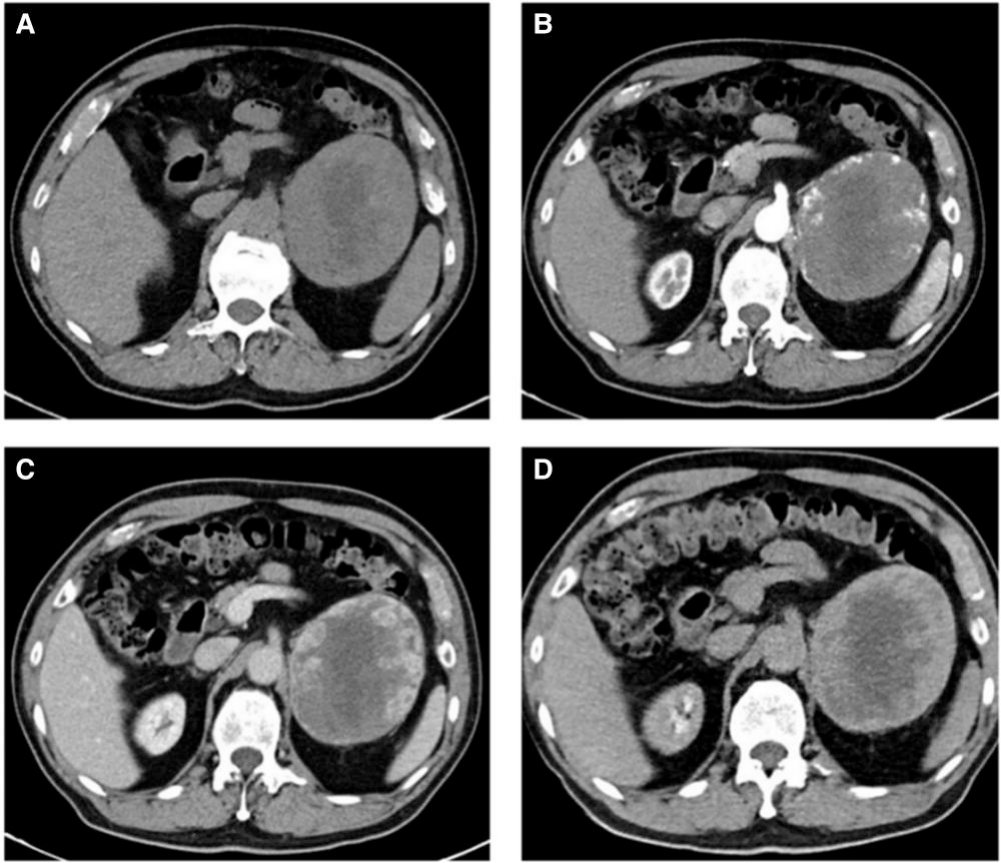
Contrast-enhanced computed tomography demonstrating typical centripetal progressive enhancement and central necrosis. (A) Unenhanced phase; (B) arterial phase demonstrating peripheral nodular enhancement; (C) venous phase showing centripetal filling; (D) delayed phase exhibiting progressive central enhancement.
